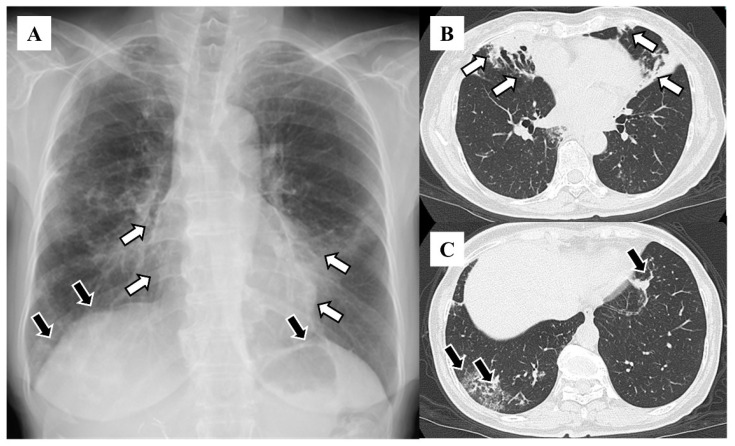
Vague cardiac silhouette on chest X-ray (A) and high-resolution computed tomography (B). White arrows indicate the same lesions within the patient. Vague diaphragm on chest X-ray (A) and high-resolution computed tomography (C). Black arrows indicate the same lesions within the patient.
